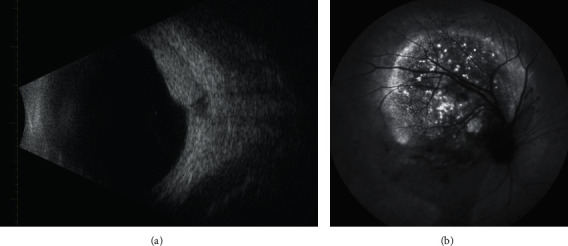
Examples of patients with LM seen on FFA and eye B ultrasonic. Abbreviations: LM: liver metastasis; FFA: fluorescence fundus angiography.
